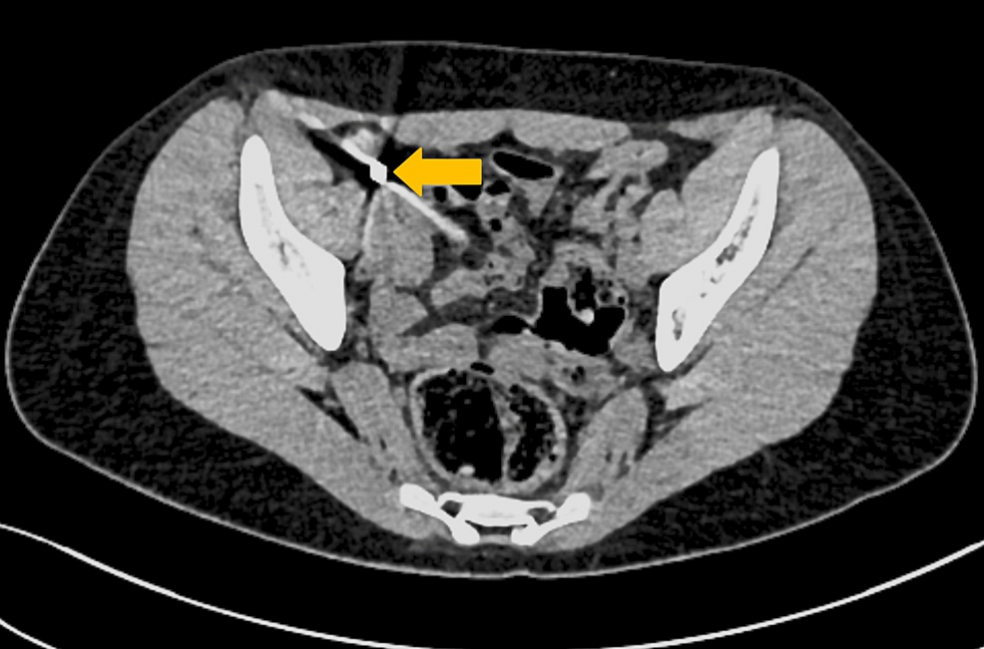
An abdominal low-dose computed tomography showing two magnetic balls in the lumen of the appendix.
